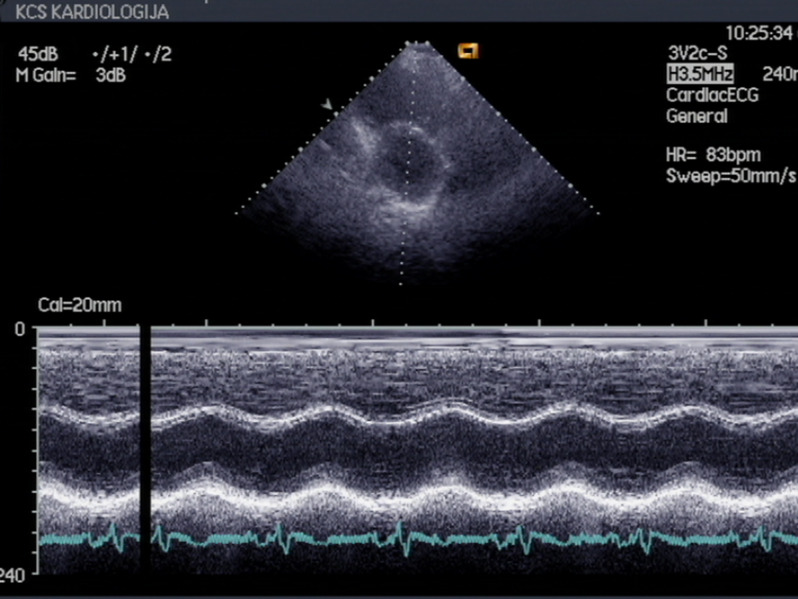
Transthoracic echocardiography shows left ventricle in parasternal short axis view with paradoxical movements of the septum
